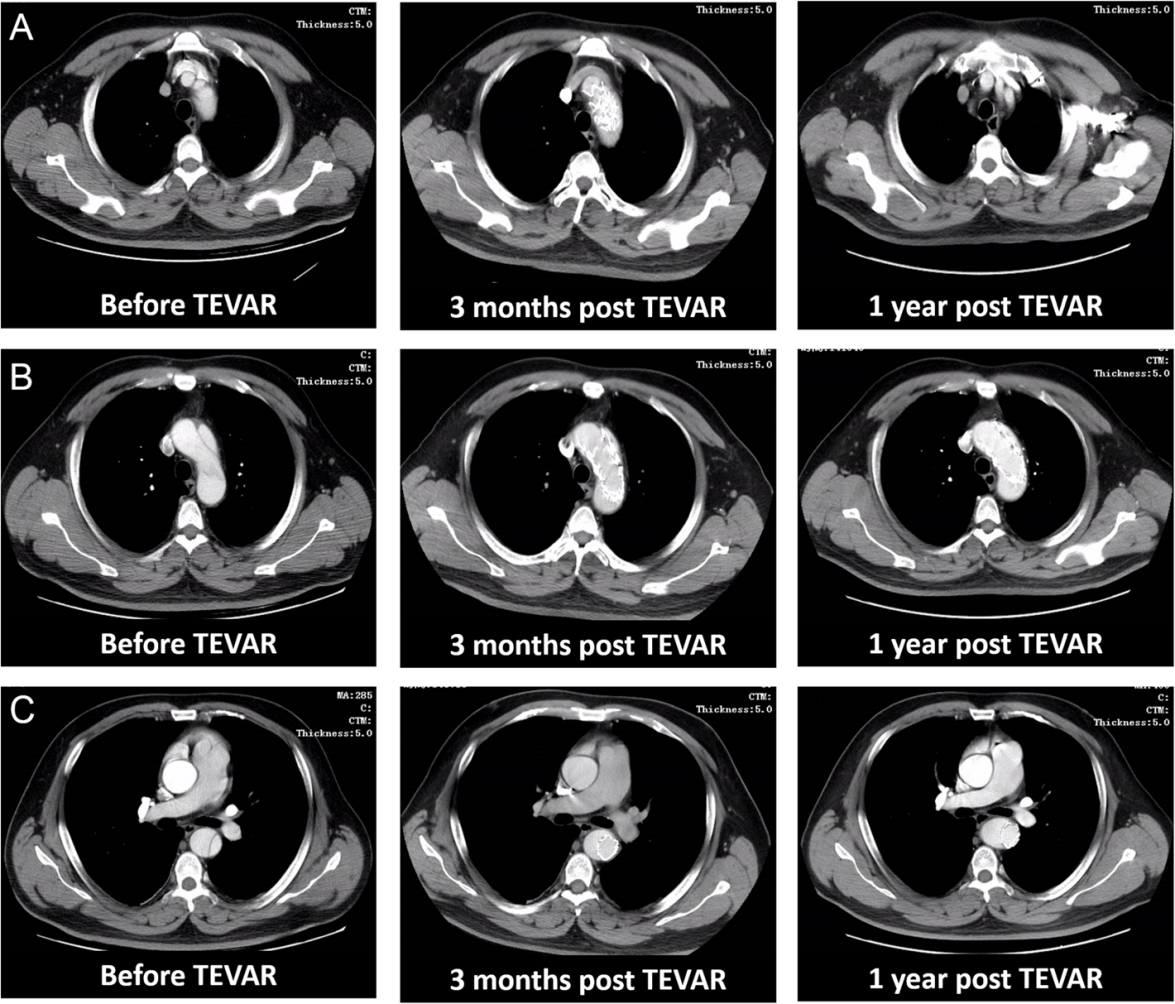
CT image of patients before and after TEVAR surgery with new blood flow regulators. a) Preoperative image of aortic arch branches showed the aortic dissection of a patient involving the beginning of the left subclavian artery (left panel). The centre image demonstrates the coverage of the stent graft 3 months after TEVAR, and the 12-month scan shows continuous blood flow in the aortic arch branches. b) The imaging of the aortic arch before surgery showed aortic dissection involving the anterior aortic arch wall (left panel). CT images 3 and 12 months after TEVAR surgery showed that aortic dissection was resected and corrected. c) The scan for pulmonary artery bifurcation before and after TEVAR
